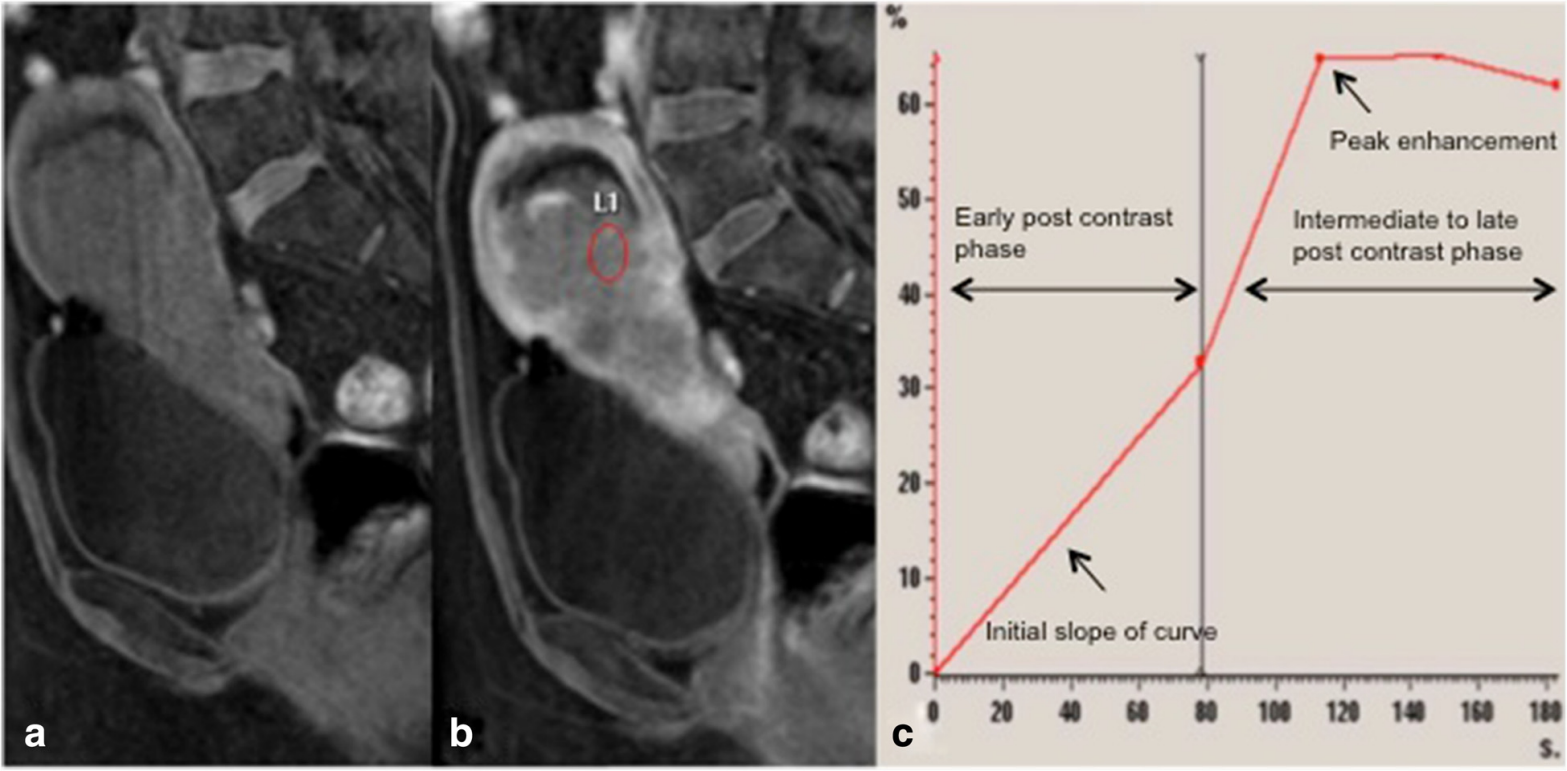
Enhancing endometrial carcinoma and type 3 malignant curve. a demonstrates the pre-contrast sagittal MRI through the uterus, following contrast at a 72 second acquisition, there is enhancement of endometrial soft tissue (b), note that the tumour is less enhancing than the adjacent myometrium and invasion by the tumour can clearly be seen. A region of interest (red circle) has been placed over the tumour and a TCI created (c), this shows a type 3 malignant curve.
